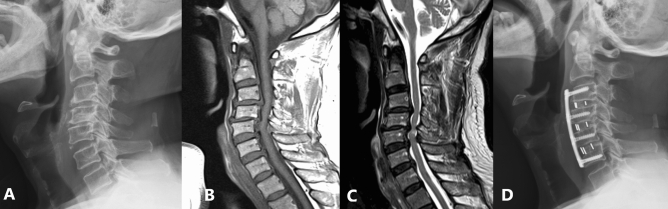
An 80-year-old male had degenerative cervical myelopathy from C3 to C6. Preoperative MRI T1W and T2W images shows spinal cord compression from C3 to C6 and cord edema at C4–C5. (a–c) He underwent three-level ACDF from C3 to C6 using four PEEK cages and one long anterior plate. Postoperative lateral cervical spine radiography at 1 year showed successful fusion from C3 to C6, maintenance of intervertebral heights, stable C2–C7 Cobb angle and improved C2–C7 SVA. (d) He had clinical improvement and smooth postoperative follow-up until 4 years later, having right middle cerebral artery territory infarction with left hemiparesis.
